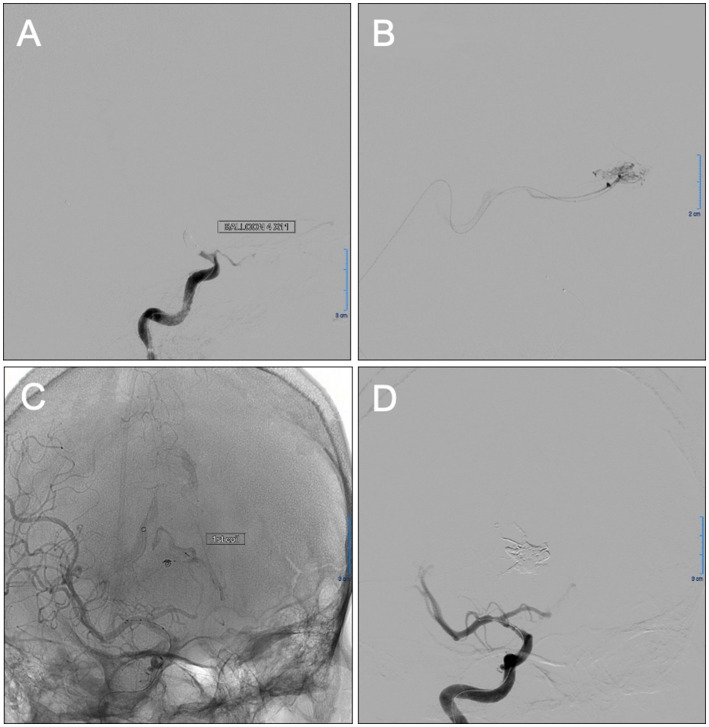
Intraoperative images showing planning and completion of the transvenous Onyx embolization of the diffuse right frontal arteriovenous malformation (AVM). Balloon test occlusion of the anterior cerebral artery A1 segment (A) was done during embolization for proximal vascular control of the AVM nidus flow. After superselective venous catheterization of the straight sinus, deep venous system, and right frontal AVM nidus, superselective runs (B) showed nidus configuration and no significant collateral reflux. Unsubstracted radiograph (C) shows proper placement of aneurysm coil proximal to the nidus performed under fluoroscopic guidance to reduce venous reflux of Onyx (pressure cooker technique). Oblique digital subtraction angiography (DSA) view of right internal carotid injection post-embolization (D) showed complete obliteration of the AVM nidus.
